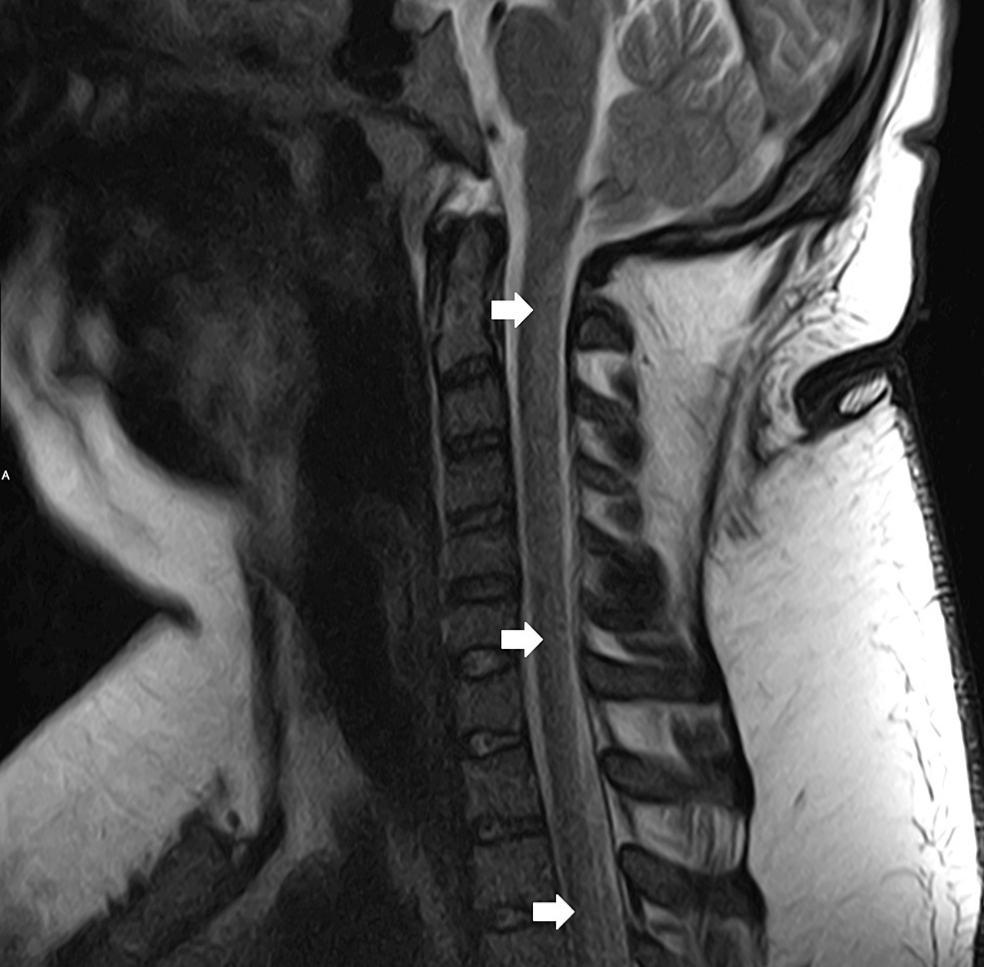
T2-weighted MRI of the cervical spine showed diffuse central and posterior cervical spinal cord signal abnormality, shown at arrow tips, consistent with subacute combined degeneration.
